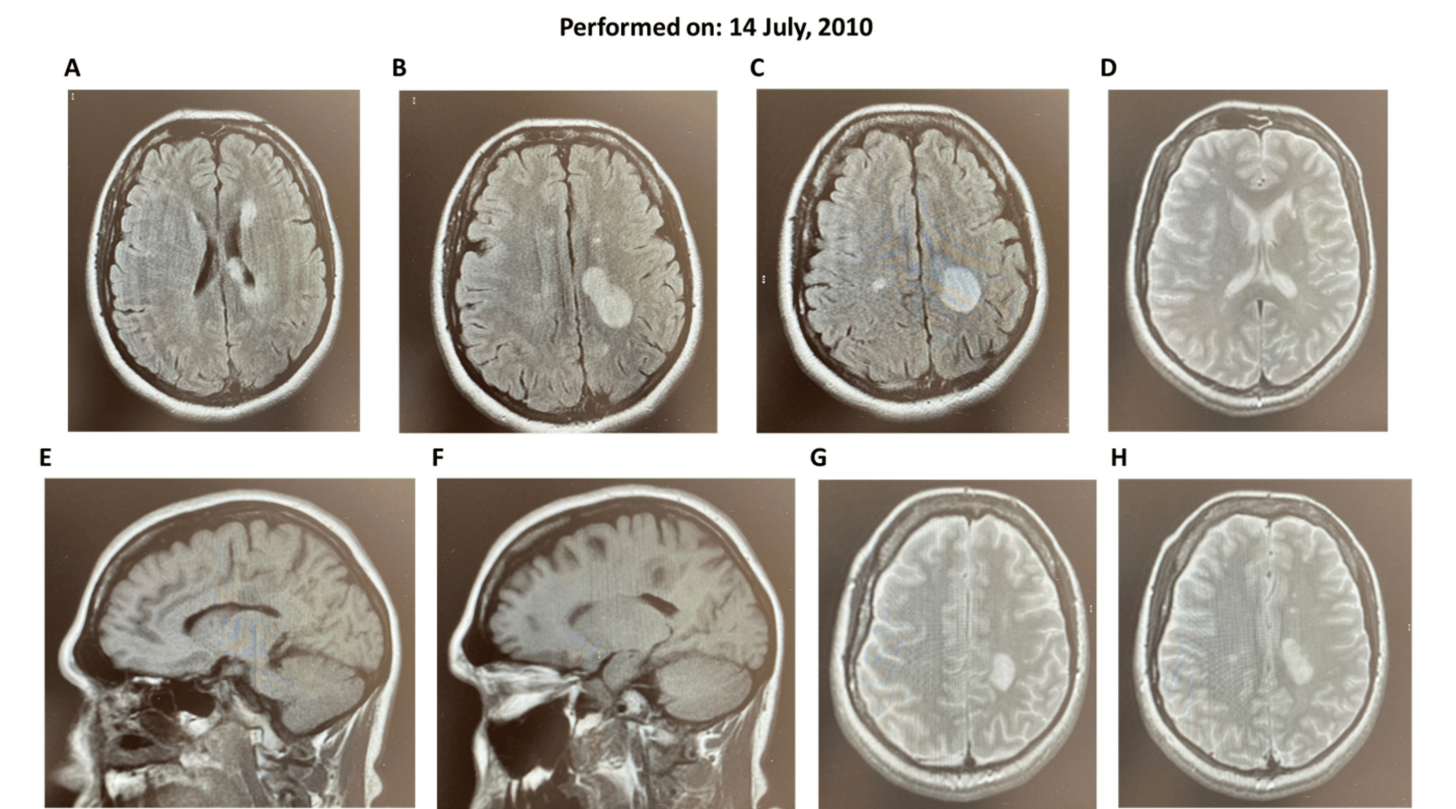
(A-H) Initial cranial magnetic resonance imaging scans for Case 1
